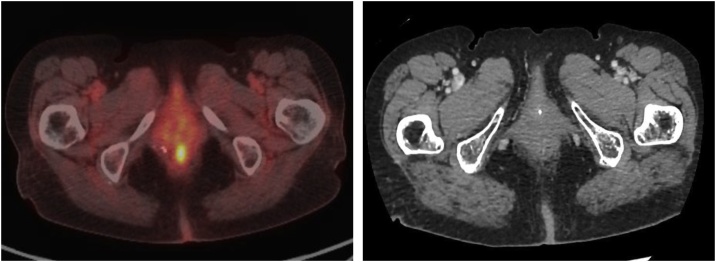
Positron emission tomography-computed tomography findings Accumulation of ^18^F-fluorodeoxyglucose is visible in a mass at the anorectal junction.
